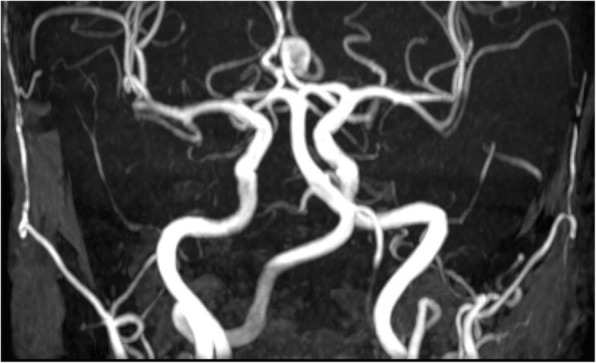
Computed tomography angiogram (CTA) of the patient demonstrating the anterior communicating artery anuerysm
